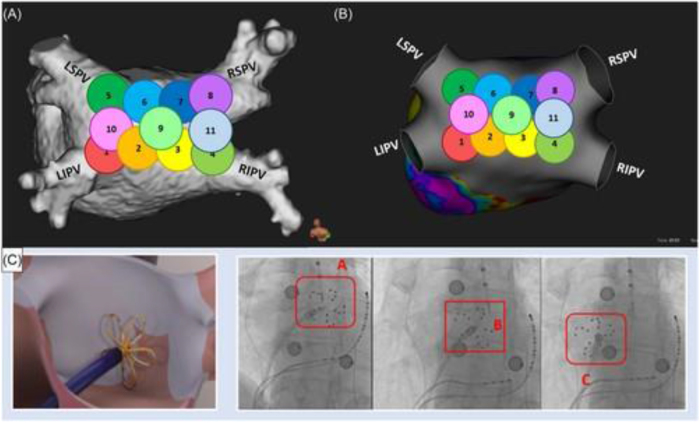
Following PVI, LAPW isolation was performed at the operator’s discretion using PFA to create continuous lesions linking the floor, roof, and inner wall of the LAPW, with additional ablation applied at carina sites when necessary. Panel (A) shows a CT reconstruction of the left atrium, Panel (B), a 3D-mapping reconstruction, and Panel (C), a fluoroscopic visualization of the LAPW ablation process.
